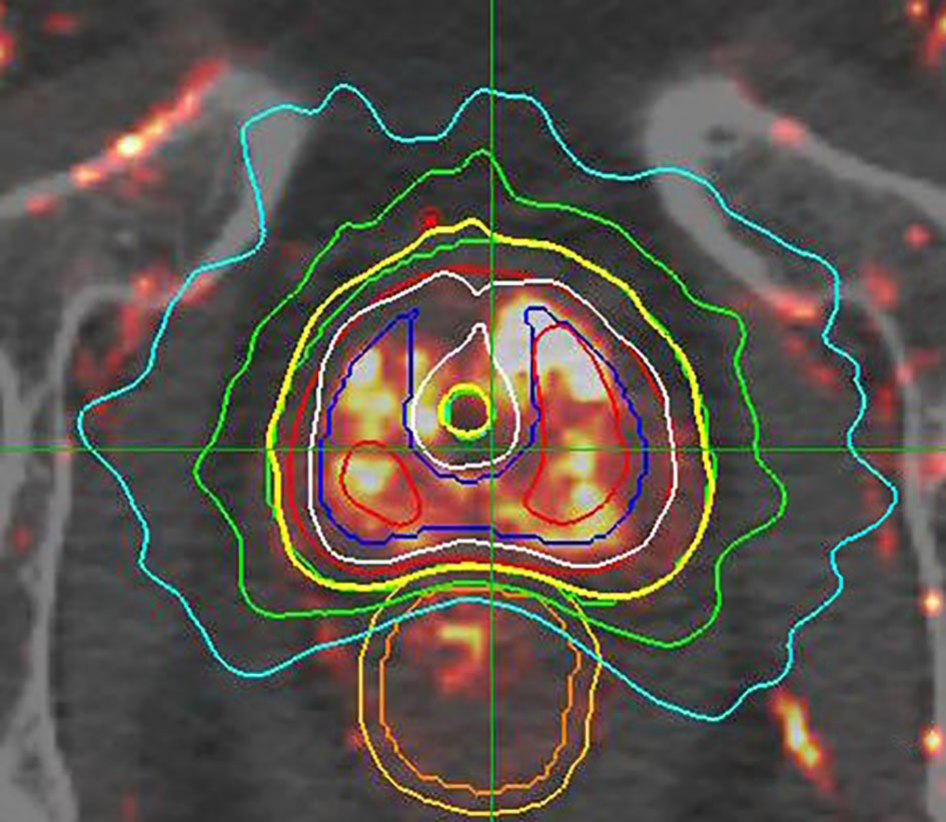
Sample HDR-like treatment plan, with contours and isodose display: This image set consists of a DCE enhanced MRI, superimposed over a standard planning CT image. The prostate GTV is contoured in red. The asymmetrically expanded PTV is contoured in green, revealing a 2 mm GTV to PTV expansion on the right (lesser involved side); a 5 mm GTV to PTV expansion on the left side (heavier involvement and with Gleason 7 disease); with manual “shaving” of GTV to PTV expansion down to zero mm adjacent to the rectum. This plan was constructed before the advent of SpaceOAR. This case is prescribed to 3800cGy/4 fractions, displayed by the yellow isodose line, with extreme conformality around the underlying green PTV contour. Additional isodose information: 125% = white, 150% = red, 75% = green, and 50% = aqua. NOTE that the yellow prescription isodose line touches, but does not breach, the outer rectal wall, and also has a central dip to relatively spare the urethra, while the 75% green isodose line touches but does not breach the rectal mucosa, defined as a 3 mm contraction from the outer rectal wall. This design morphology concentrates the greatest dose in the peripheral zone of the most heavily involved left lobe, with wider coverage margins adjacent to that region.
